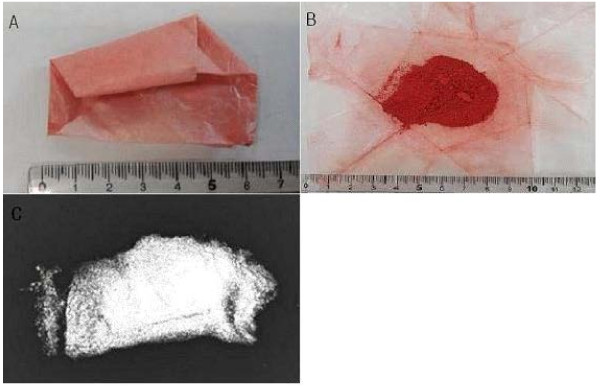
Cinnabar patient had taken with talisman ash. (A, B) Cinnabar powder (C) A simple X-ray of cinnabar powder.
